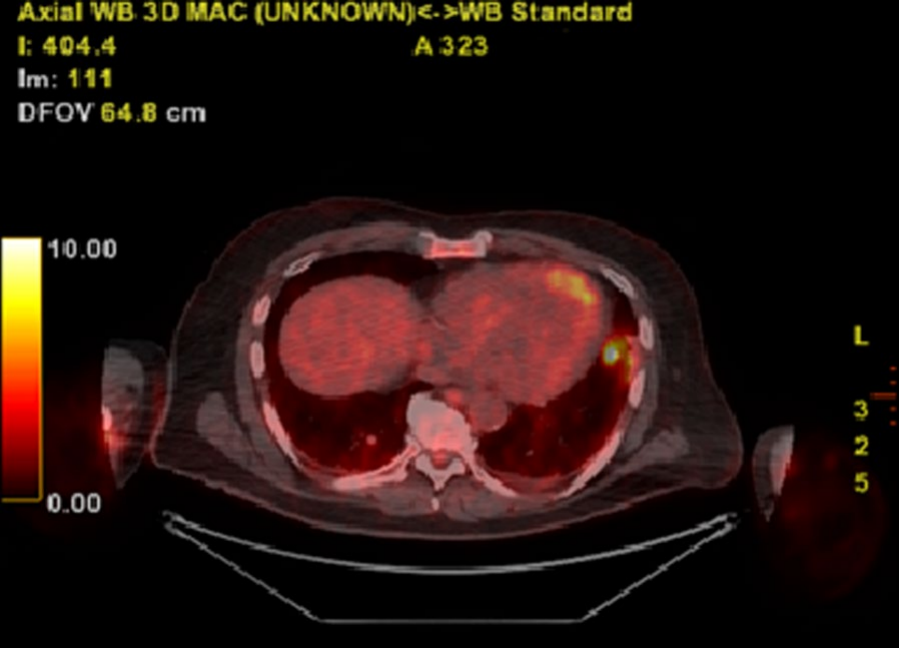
PET-CT scan showing increased FDG uptake from the lingula lesion (SUVmax: 13)
